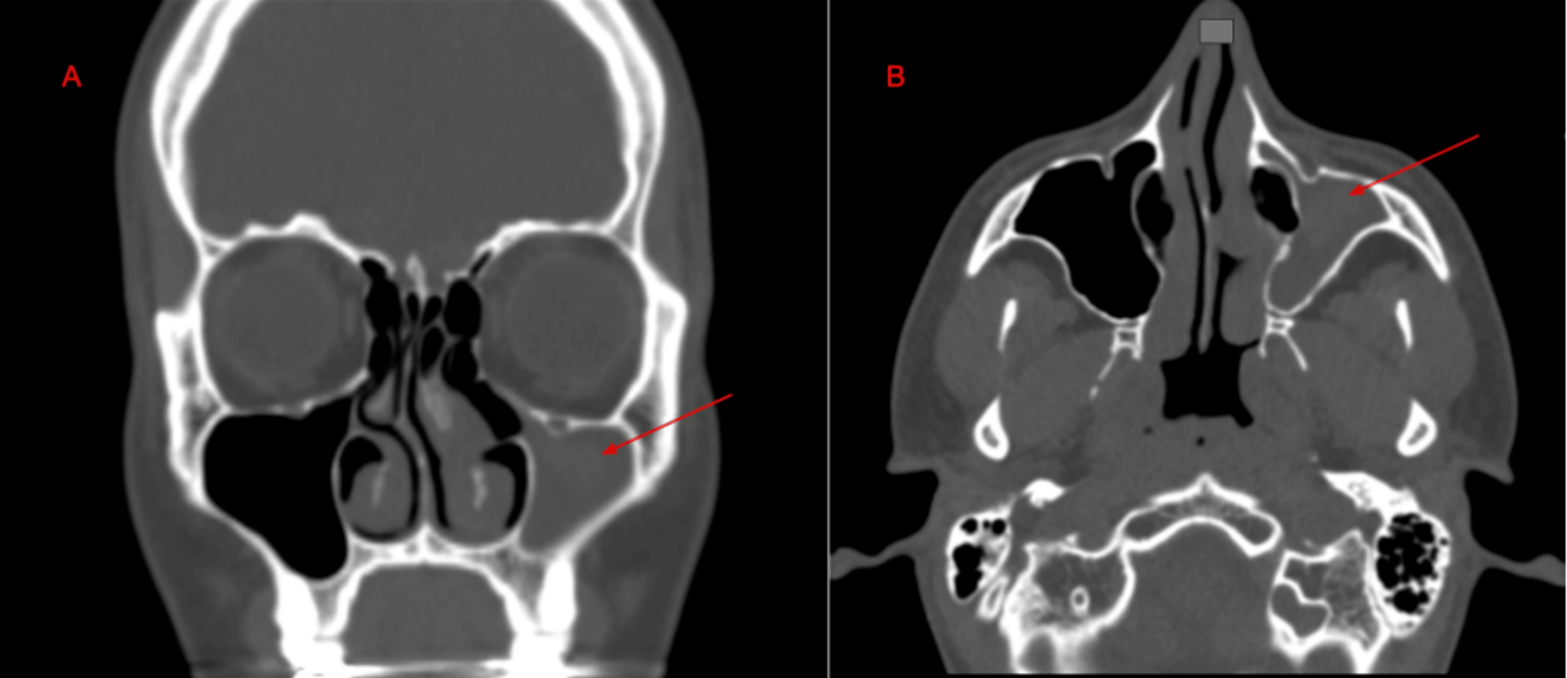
Non-contrast CT imaging of the paranasal sinuses demonstrating left maxillary sinus opacification and associated findings. Coronal (A) and axial (B) views of a non-contrast CT scan of the paranasal sinuses showing complete opacification of the left maxillary sinus (red arrows). Additional findings include atelectasis of the left uncinate process and inward bowing of the medial maxillary sinus wall.
